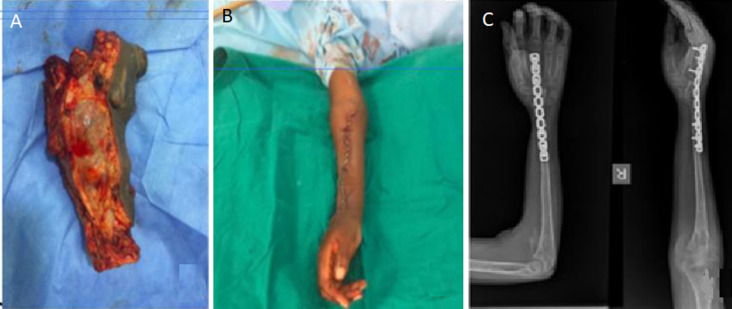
post en bloc resection and wrist fusion: A) macroscopic appearance of the resected mass with fluid-fluid like lobules; B) post-operative wound whereby primary wound closure was achieved; previous scars with relapses were included in the en bloc tumor resection to ensure adequate surgical margins; C) radiography of the right forearm with centralized ulnar
